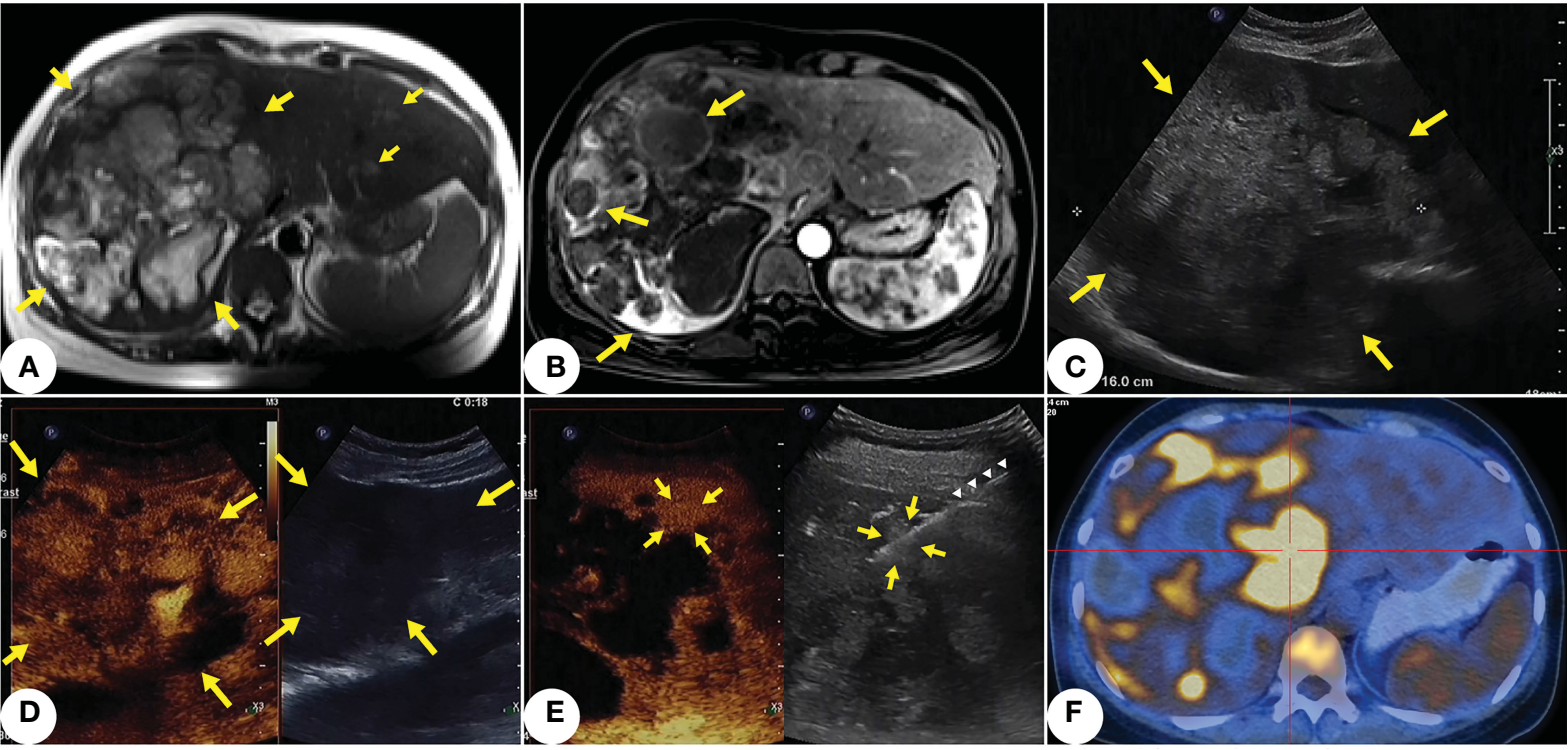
Primary hepatic angiosarcoma (PHA) in a 42-years-old woman. (A) T2-weighted axial MRI shows multiple variable-sized lesions with bright T2 signal intensity are located in both hepatic lobes. Mass lesions of PHA reveal a markedly heterogeneous architecture, with focal areas of high intensity along with septum-like or rounded areas of low intensity (indicated by yellow arrows). (B) Some lesions show annular enhancement at the edges; large masses show ruptures and hemorrhages (indicated by yellow arrows). (C) Ultrasound shows a 16cm inhomogeneous hypoechoic lesion in the right hepatic lobe (indicated by yellow arrows). Multiple masses scatter within both hepatic lobes. (D) Contrast-enhanced ultrasound shows the lesion (indicated by yellow arrows) in ill-defined, peripheral irregular isoenhancement, and necrotic and hemorrhagic areas within the tumor. (E) After selecting a target lesion with multimodal enhanced imaging, (indicated by yellow arrows), we performed core needle biopsy (indicated by white triangles) under the guidance of contrast-enhanced ultrasound. (F) PET-CT shows multiple hypermetabolism lesions with retroperitoneal lymph node metastasis in the liver.
